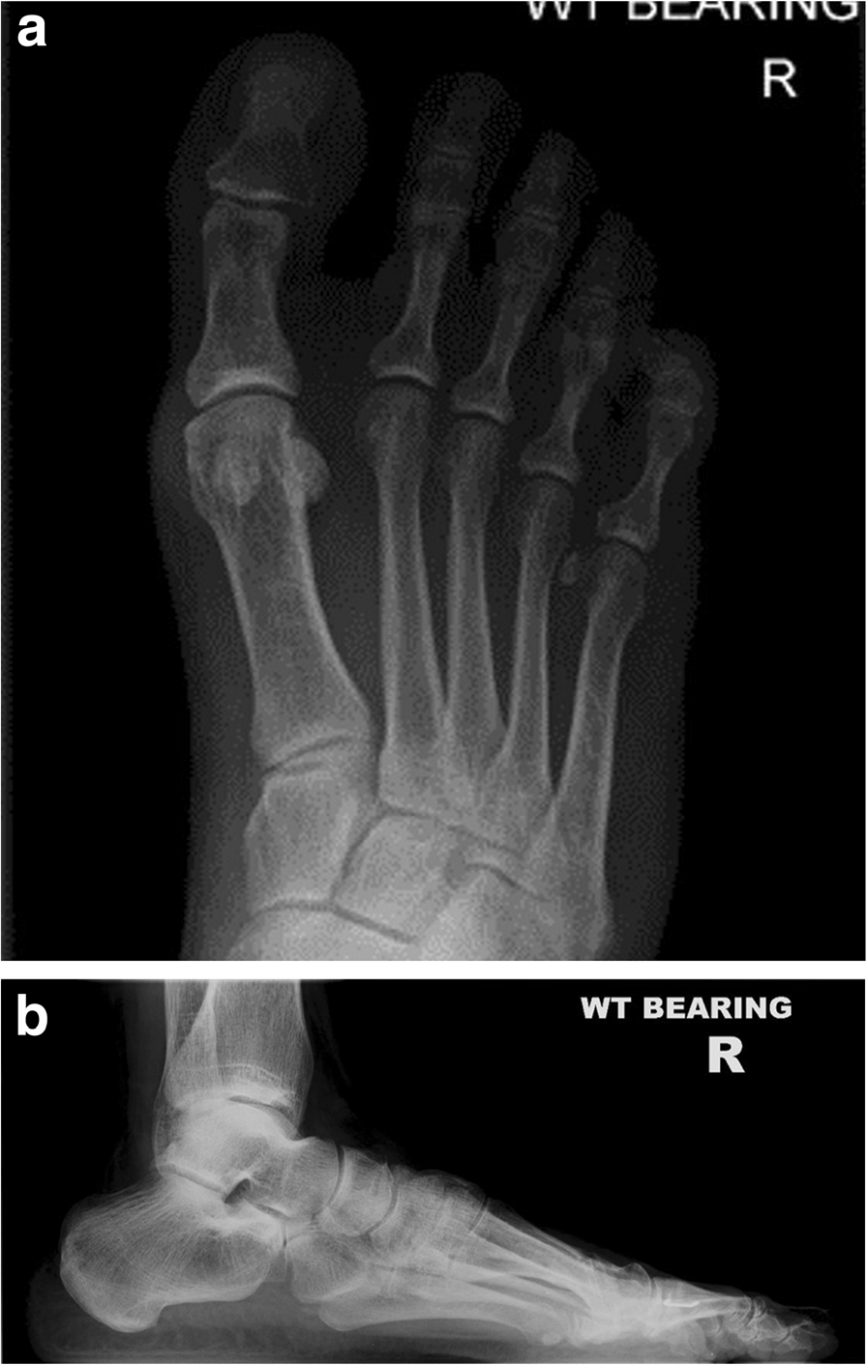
a and b Pre-operative AP and lateral radiographs
